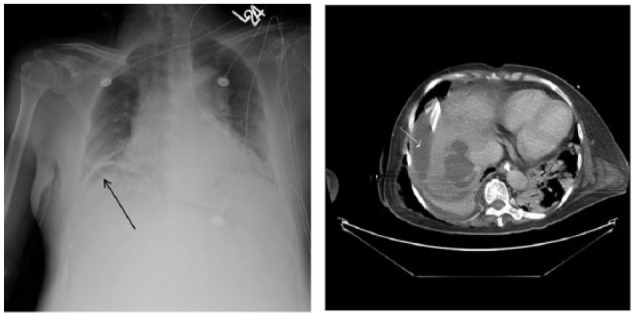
(A) Chest x-ray demonstrating a crescentic lucency underneath the right hemidiaphragm concerning for free air within the abdomen (black arrow). (B) Large right liver abscess (white arrow).
